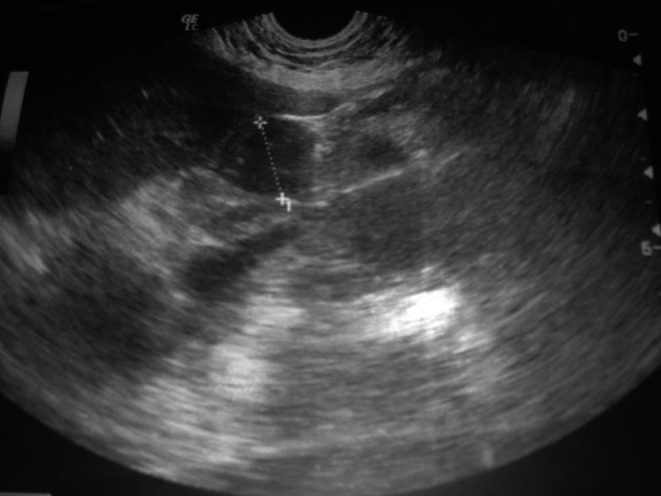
Ultrasound: hypoechogenous, nodular formation in the pancreatic body
